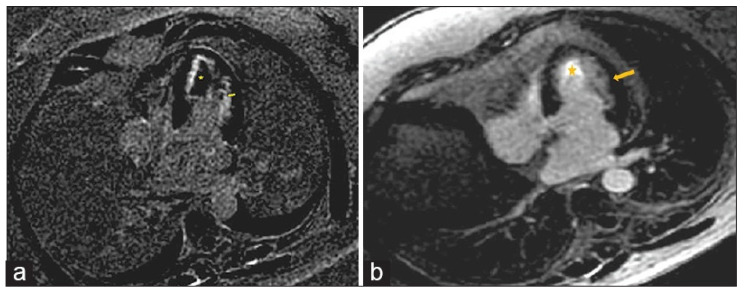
(a) Cardiac magnetic resonance imaging, apical four-chamber, post-contrast late gadolinium enhancement images showing extensive subendocardial fibrosis (arrow) and left ventricle mural thrombus (star). (b) Cardiac magnetic resonance imaging, apical four-chamber, follow-up post-contrast late gadolinium enhancement images showing significant improvement of subendocardial fibrosis (arrow) and resolution of left ventricle mural thrombus (star). Reproduced from: Taskesen, T., et al. (2022) [62]. Licensed under CC BY-NC 4.0 (https://creativecommons.org/licenses/by-nc/4.0/ accessed on 15 December 2025).
